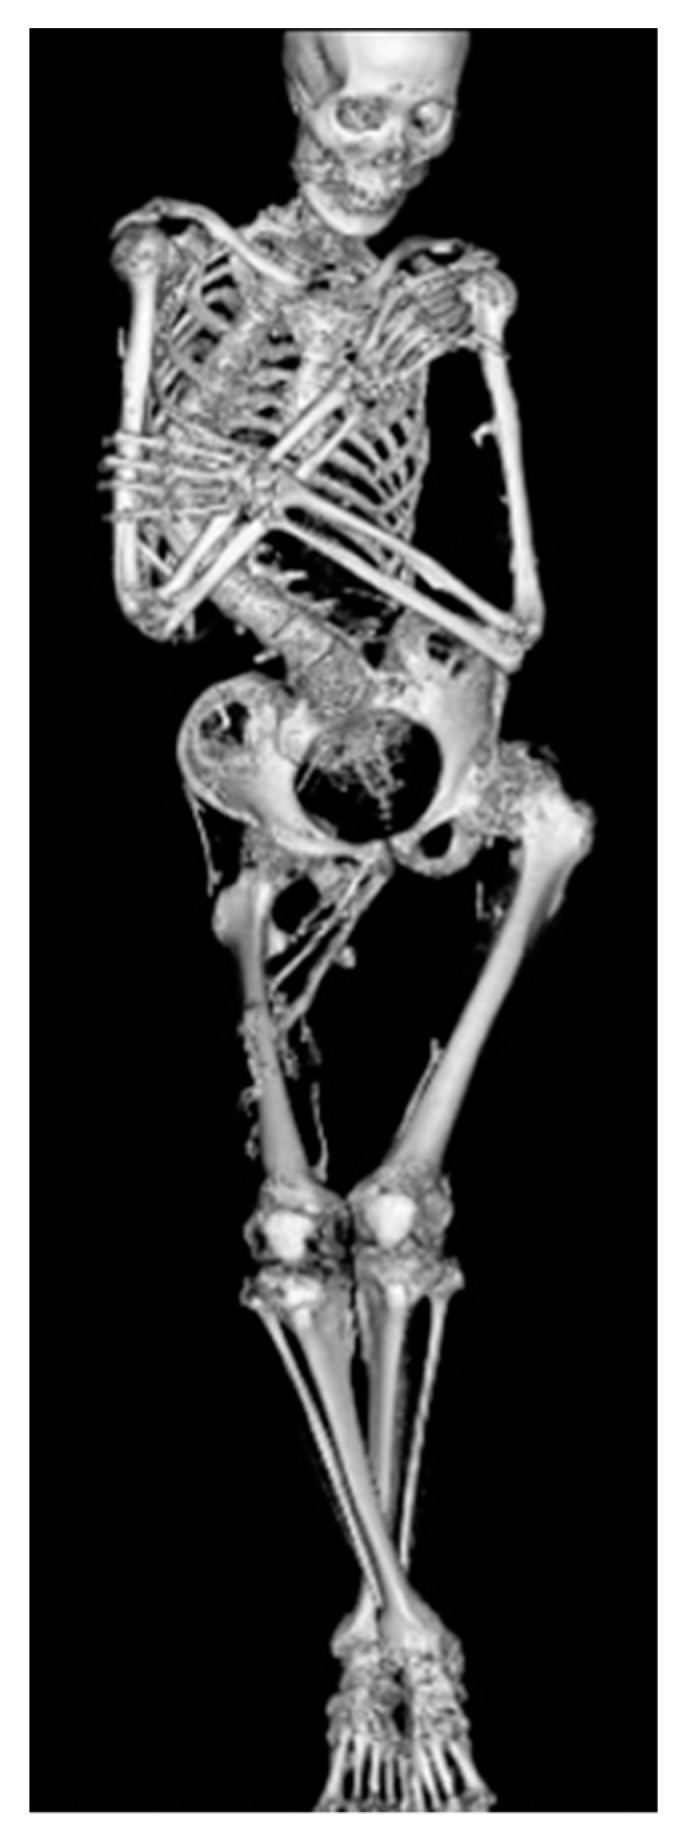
Whole body computed tomography imaging of 26-year-old male with FOP showing severe rigid scoliosis and bilateral hip joint contractures due to heterotopic ossifications across the joints.
